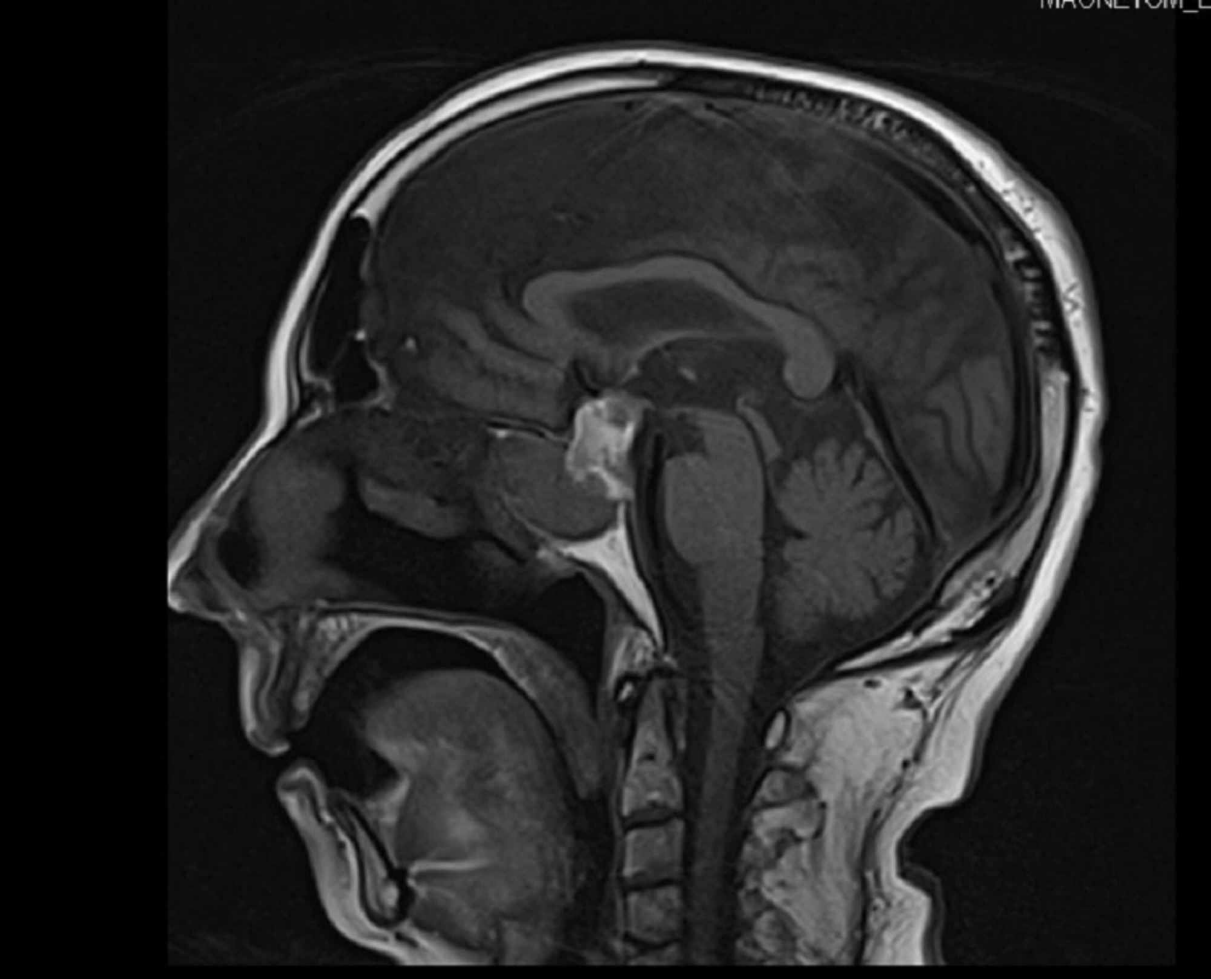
MRI brain showing pituitary macroadenoma with hemorrhagic areas. MRI: magnetic resonance imaging
